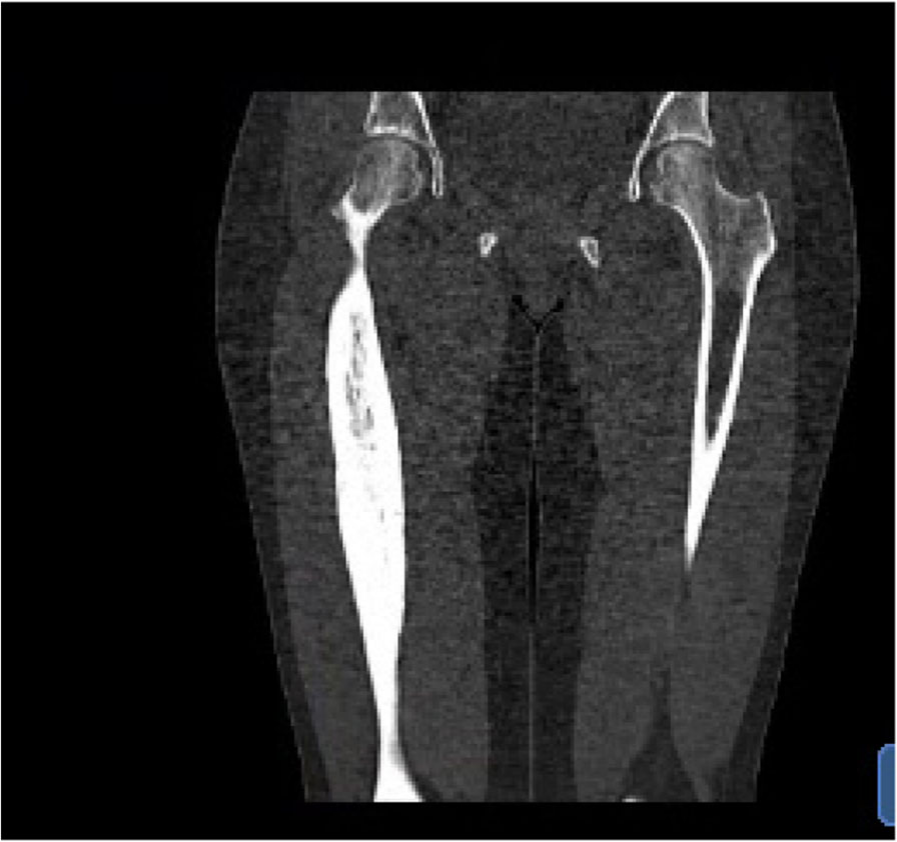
Computed tomography of right femoral shaft. Computed tomography scan showing cortical thickening of the patien’s right femoral shaft
